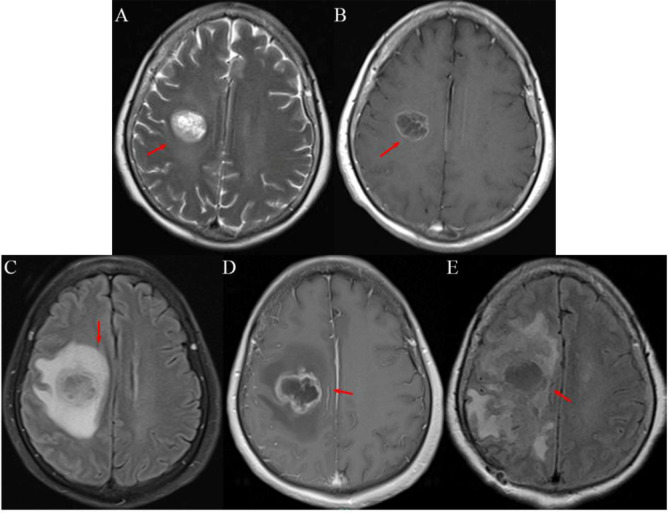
(A, B) MRI (November 26, 2024). (A) T2 TSE image showing the lesion with surrounding edema (arrow). (B) Contrast-enhanced T1-weighted image showing ring enhancement, with lesion measuring 2.6 cm × 2.2 cm (arrow). (C, D) MRI (January 21, 2025). (C) T2 FLAIR image showing further enlargement of the lesion with markedly increased peritumoral edema (arrow). (D) Contrast-enhanced T1-weighted image showing lesion measuring 2.7 cm × 2.5 cm with persistent ring enhancement (arrow). (E) MRI (September 1, 2025) T2 FLAIR image showing significant tumor progression with lesion measuring 5.0 cm × 8.0 cm after glioblastoma diagnosis (arrow).
